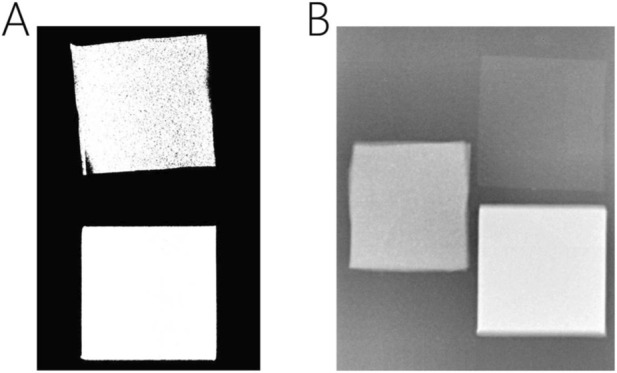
Radiopacity Test. (A) CT scan; (B) X-ray fluoroscopy.
